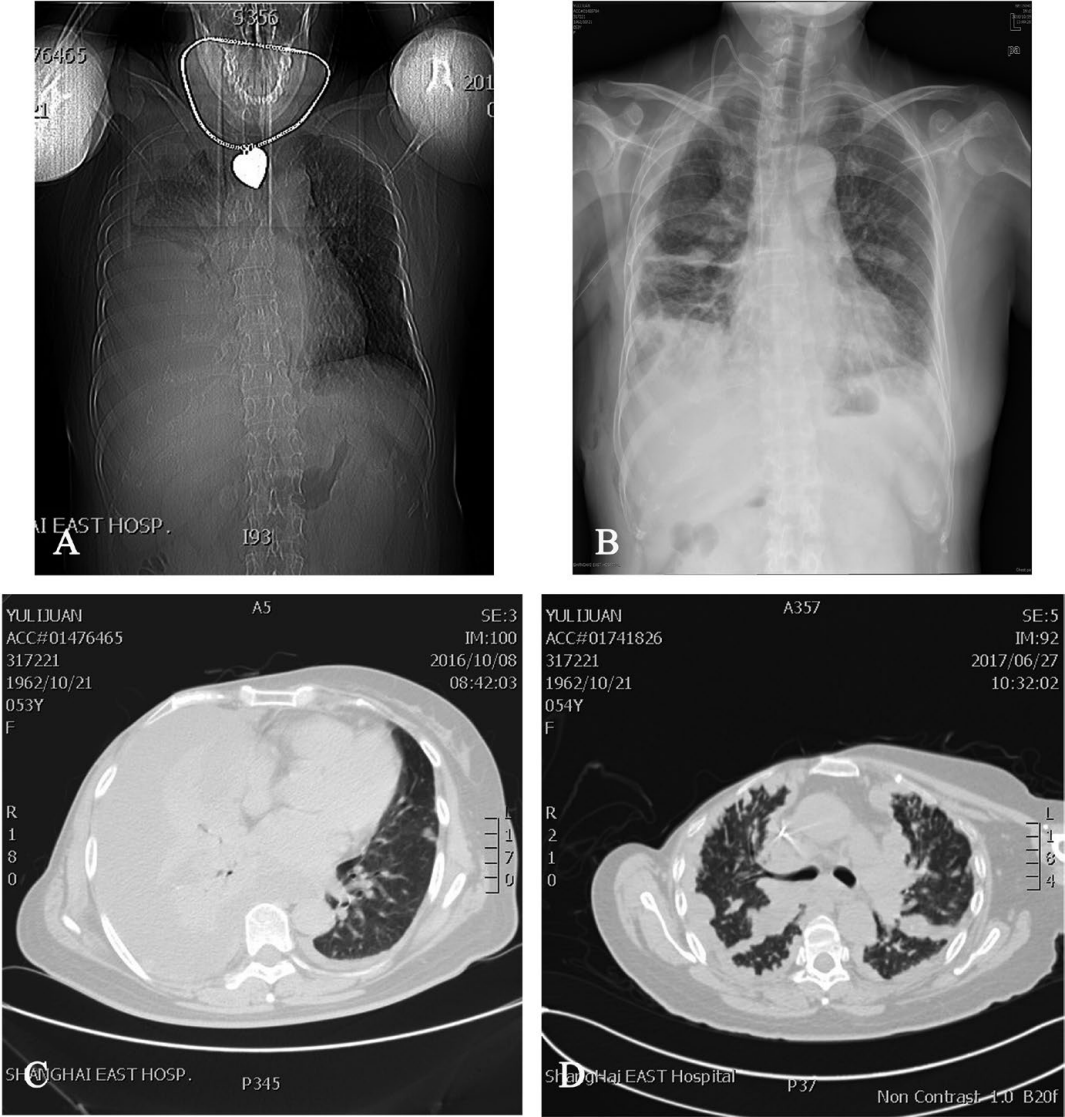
A 53‐year‐old female patient underwent IPHC by VATS and diagnosed with mucinous breast carcinoma and MPE. (A) A chest radiograph showed a large pleural effusion before treatment. (B) A chest radiograph showed the pleural effusion was cured after IPHC. (C) A CT imaging showed that a large pleural effusion before treatment. (D) A CT imaging showed that only a small pleural effusion after 9 months. IPHC, intrapleural perfusion with hyperthermic chemotherapy; VATS, video‐assisted thoracoscopic surgery; MPE, malignant pleural effusion
